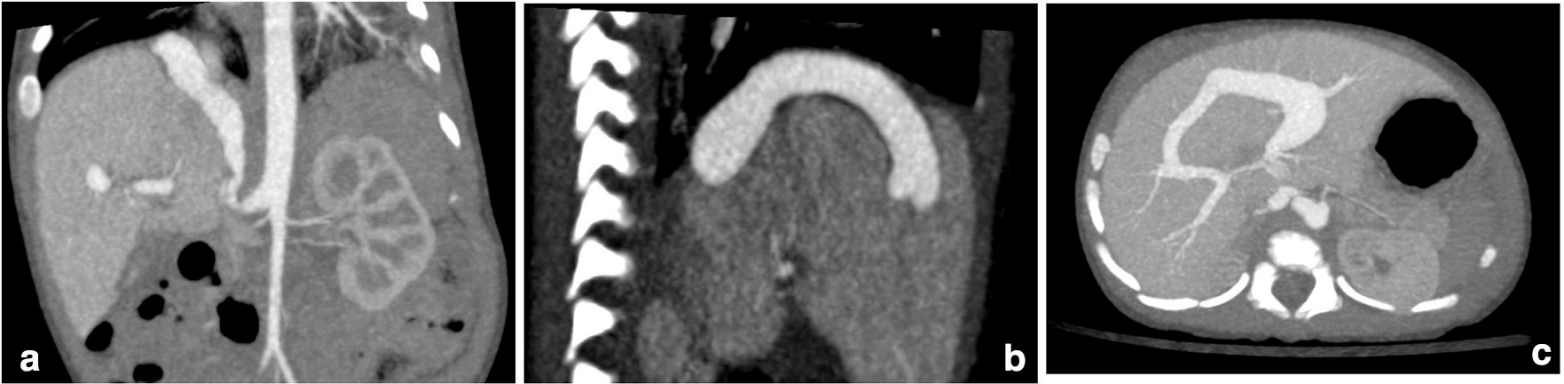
Primary CT scan showing cIAPF in coronal (a), saggital (b) and axial (c) views.
